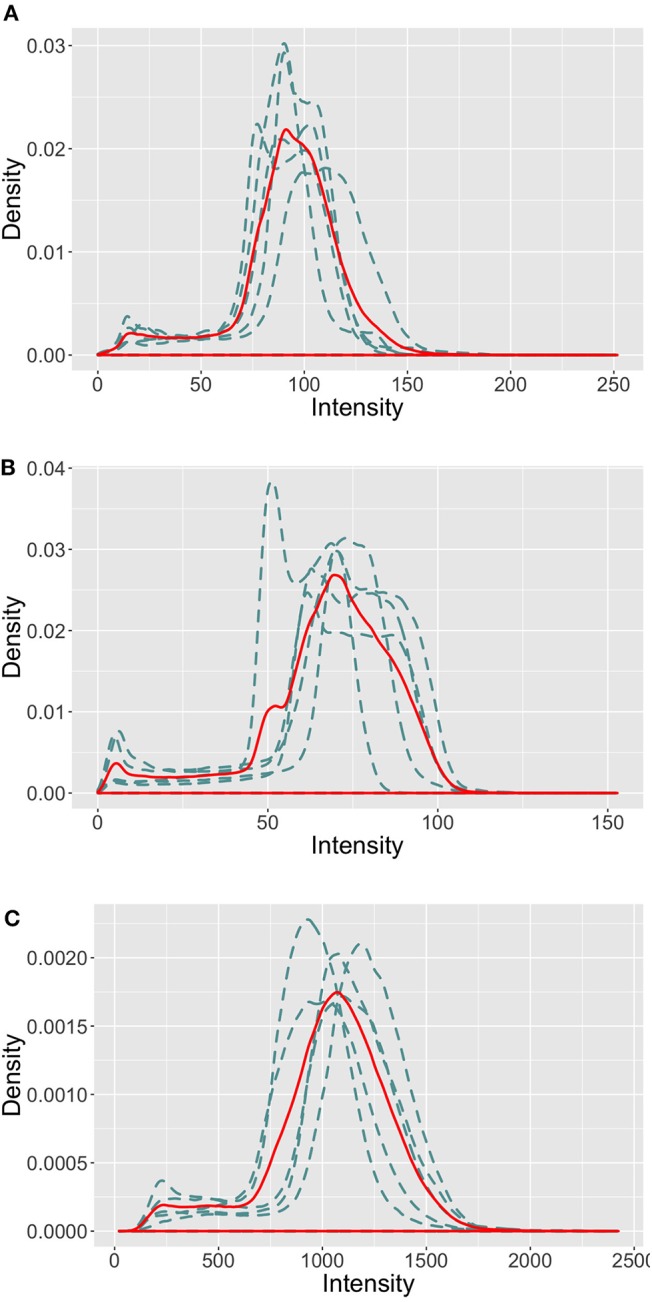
Intensity profiles in the brain area of the FLAIR images in the MICCAI 2016 train set. The blue dashed line represents the intensity distribution of each image, the red solid line represents the mean intensity distribution of the site images. (A) Site 01. (B) Site 07. (C) Site 08.
